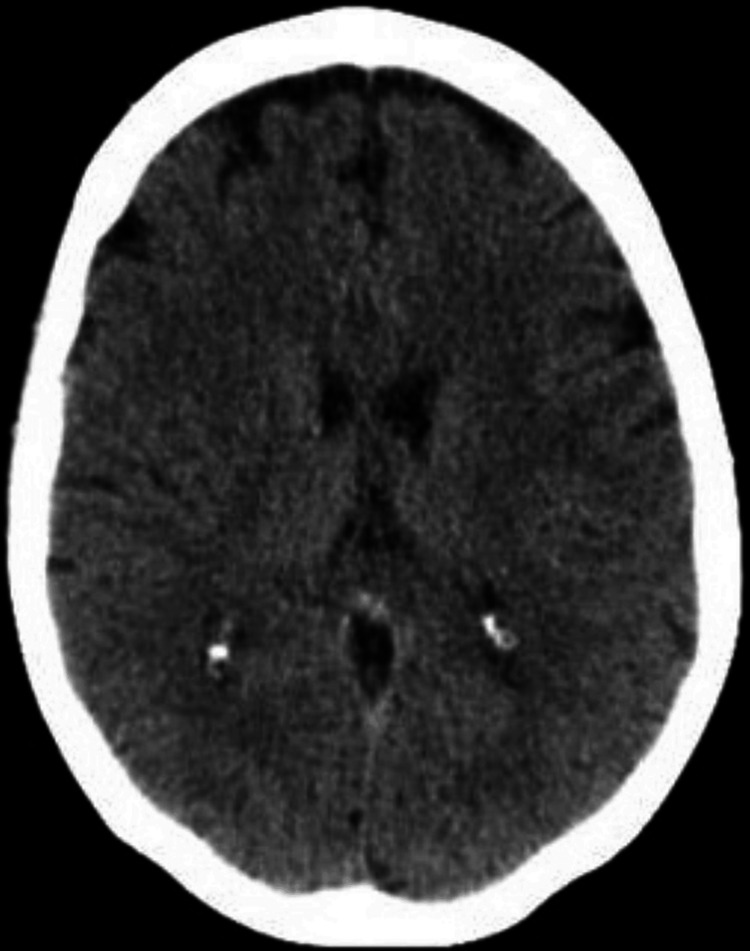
Head computed tomography showing small subcortical hypodensities
